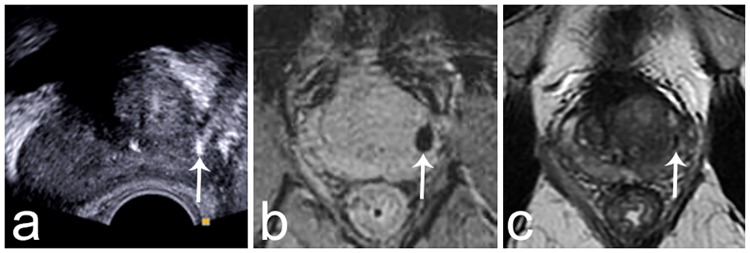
Images showing a fiducial marker implanted in the peripheral zone of the left mid-gland. A) Axial transrectal ultrasound image. The marker is markedly hyperechoic (arrow). B) Axial T2-weighted gradient echo image. The marker creates a void artifact that helps localizing it (arrow). C) Axial T2-weighted Turbo-Spin Echo image obtained at the same location as the gradient echo image. The marker is visible as a thin hyposignal (arrow).
